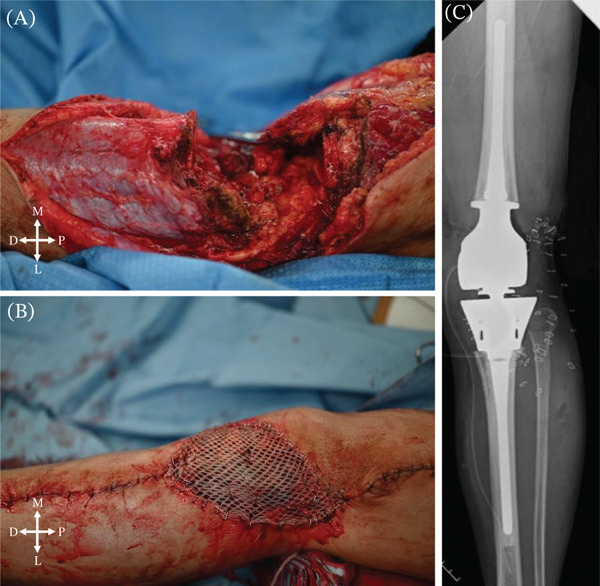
Operative procedure and postoperative radiograph. (A) An extracapsular resection including the patella was performed, followed by reconstruction using a distal femoral replacement. (B) The extensor mechanism was restored by suturing the quadriceps tendon to a gastrocnemius muscle flap including Achilles tendon, and skin grafting was carried out to complete the procedure. A postoperative radiograph is shown in (C).
